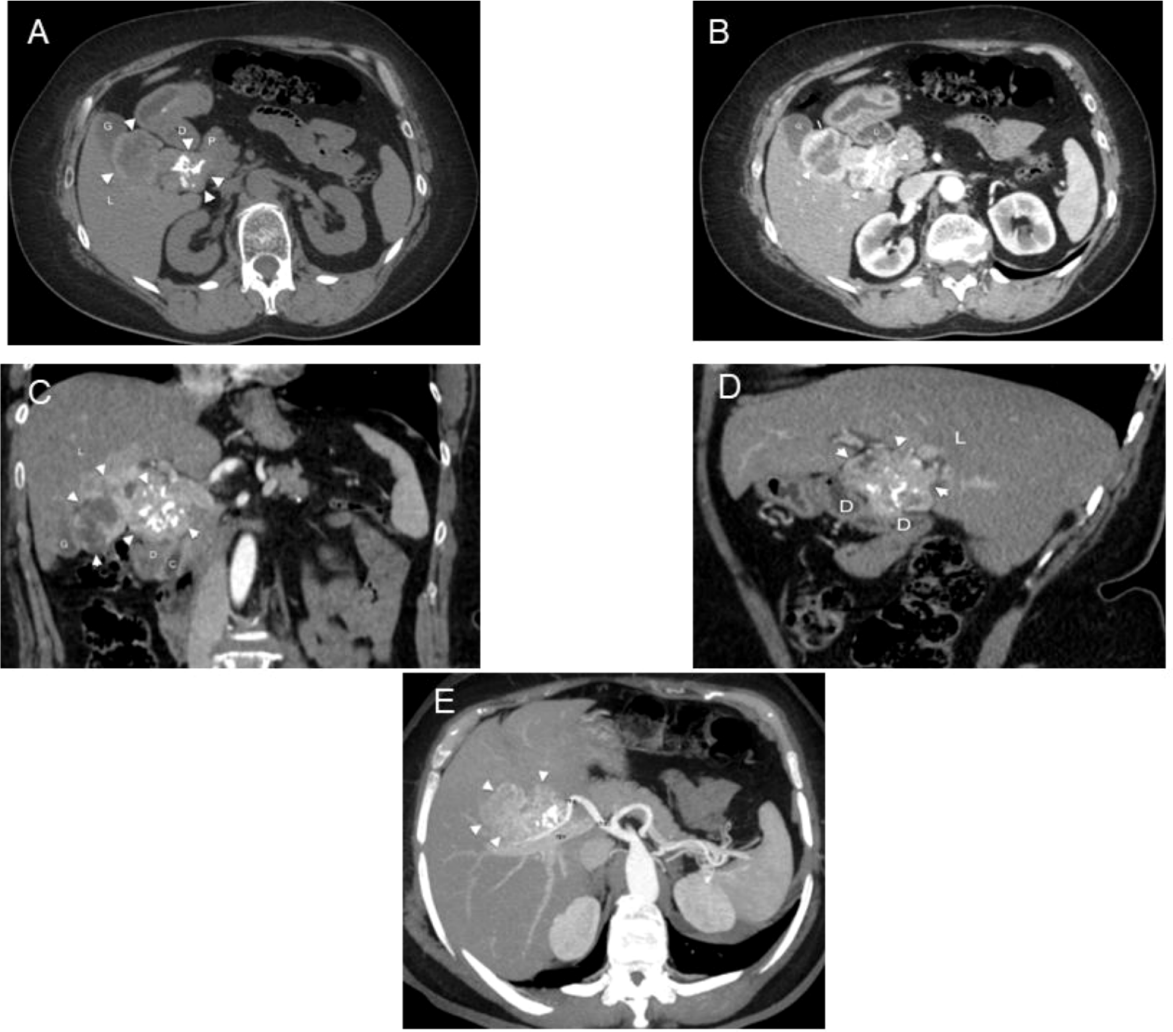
CT in a 65-year-old woman with ectopic thyroid in the hepatic hilum. (A) Unenhanced and (B) enhanced axial CT images. Dishomogeneous density and partially calcified mass (arrowheads) can be observed in the right hypochondrium between the duodenum (D), liver (L), pancreas (P), and gallbladder (G). Dishomogeneous enhancement of the mass is appreciable in the arterial phase. (C) Enhanced coronal CT image. The mass (arrowheads) has a bilobated shape and occupies the hepatic hilum, between the liver (L), gallbladder (G), duodenum (D), pancreas (P), and choledoch (C). (D) Enhanced sagittal CT image. The mass (arrowheads) pushes downward the second portion of the duodenum (D), being interposed between it and the liver (L). (E) Axial 3D MIP. The mass (arrowheads) is supplied by a branch of the right hepatic artery (rha); cha, common hepatic artery; rpv, right portal branch.
